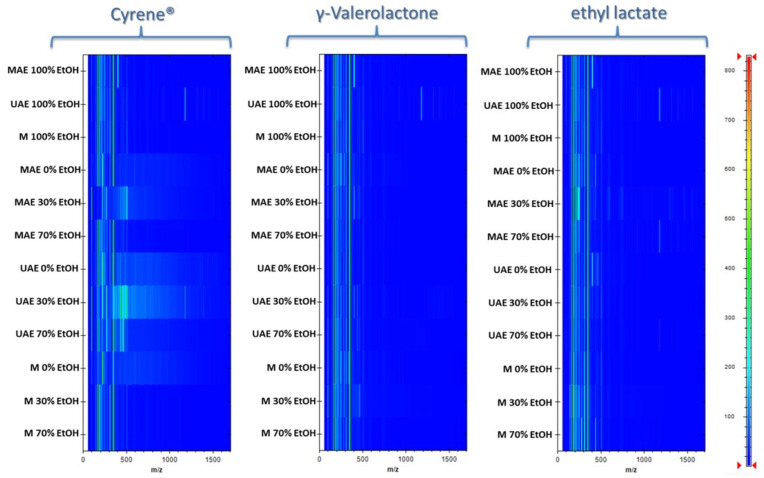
Gel-view MALDI-TOF MS spectra of rose hip extracts obtained using maceration (M), ultrasound-assisted extraction (UAE), and microwave-assisted extraction (MAE).
